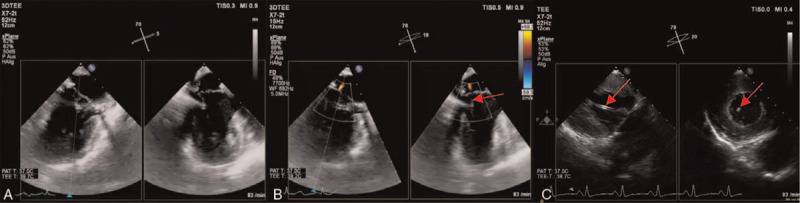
TEE of the mitral valve after NeoChord implantation. Panel A: Biplane view without a residual prolapse or flail leaflet. Panel B: Biplane view with Doppler color flow imaging revealing only a trivial residual mitral regurgitation after implant. Red arrow points to neo-chord that was attached to the posterior mitral leaflet. Panel C: Transgastric biplane long and short axis views of the left ventricle. Red arrows point to the echo-dense neo-chordae.
